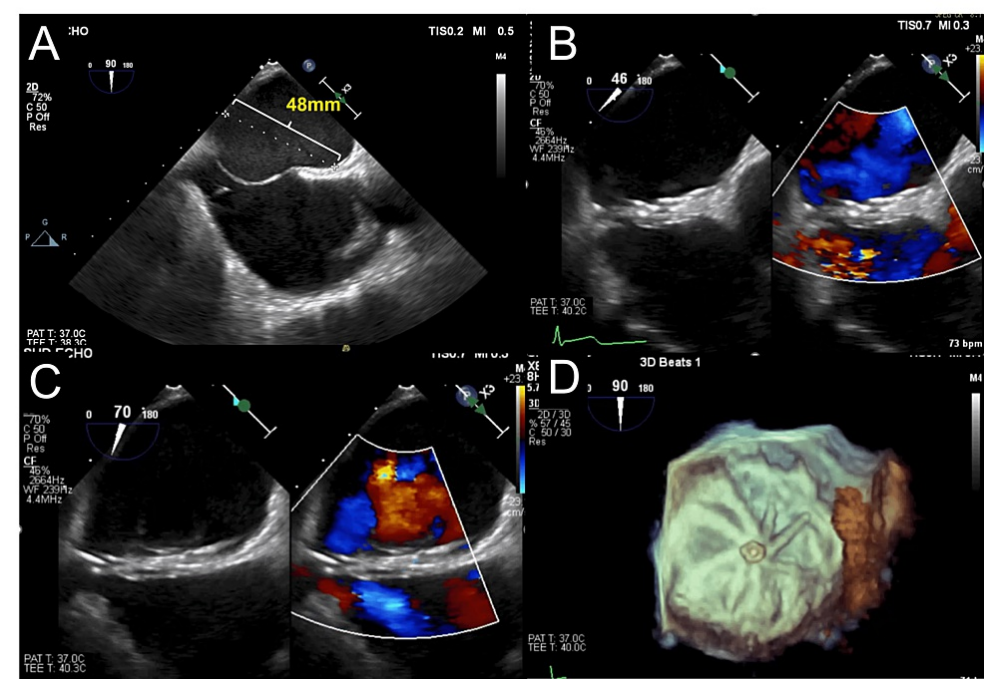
Insertion of an upsized GCA device (A) The left atrial diameter was 48 mm, allowing the insertion of an even larger GCA device. (B and C) A 44-mm GCA was implanted to cover the ASD, ASA, and PFO and to control the movement of the ASA. (D) 3D TEE image after the 44-mm GCA device implantation. 3D, three-dimensional; ASA, atrial septal aneurysm; ASD, atrial septal defect; GCA, GORE® Cardioform ASD Occluder; PFO, patent foramen ovale; TEE, transesophageal echocardiography
